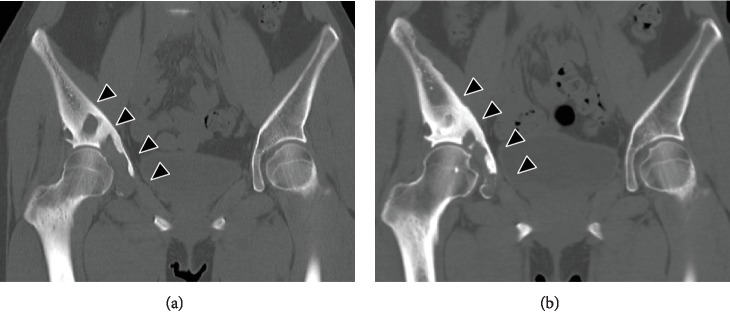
(a, b) Coronal view of computed tomography image at 10 years after the first visit revealed a partially sclerotic lesion of the ilium and acetabulum. (a) At first visit. (b) Ten years after the first visit.
